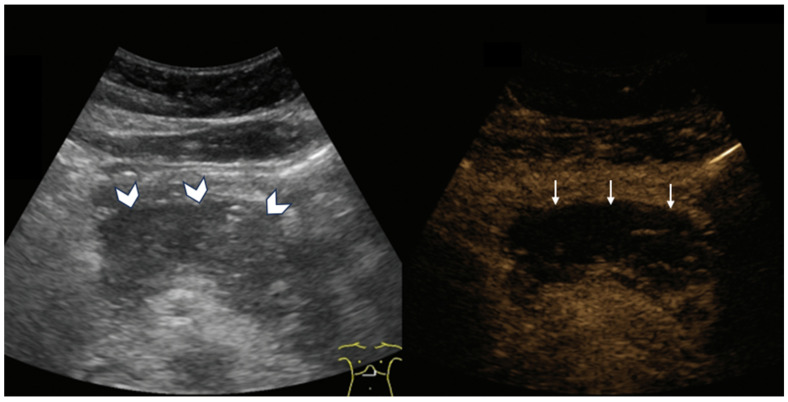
Gray scale (left) and contrast-enhanced (right) pancreatic ultrasound shows enlarged and heterogeneous pancreas (arrowhead) and non-enhancement of the almost entire pancreatic parenchyma (arrow), revealing extensive necrosis in a case of acute pancreatitis [61].
